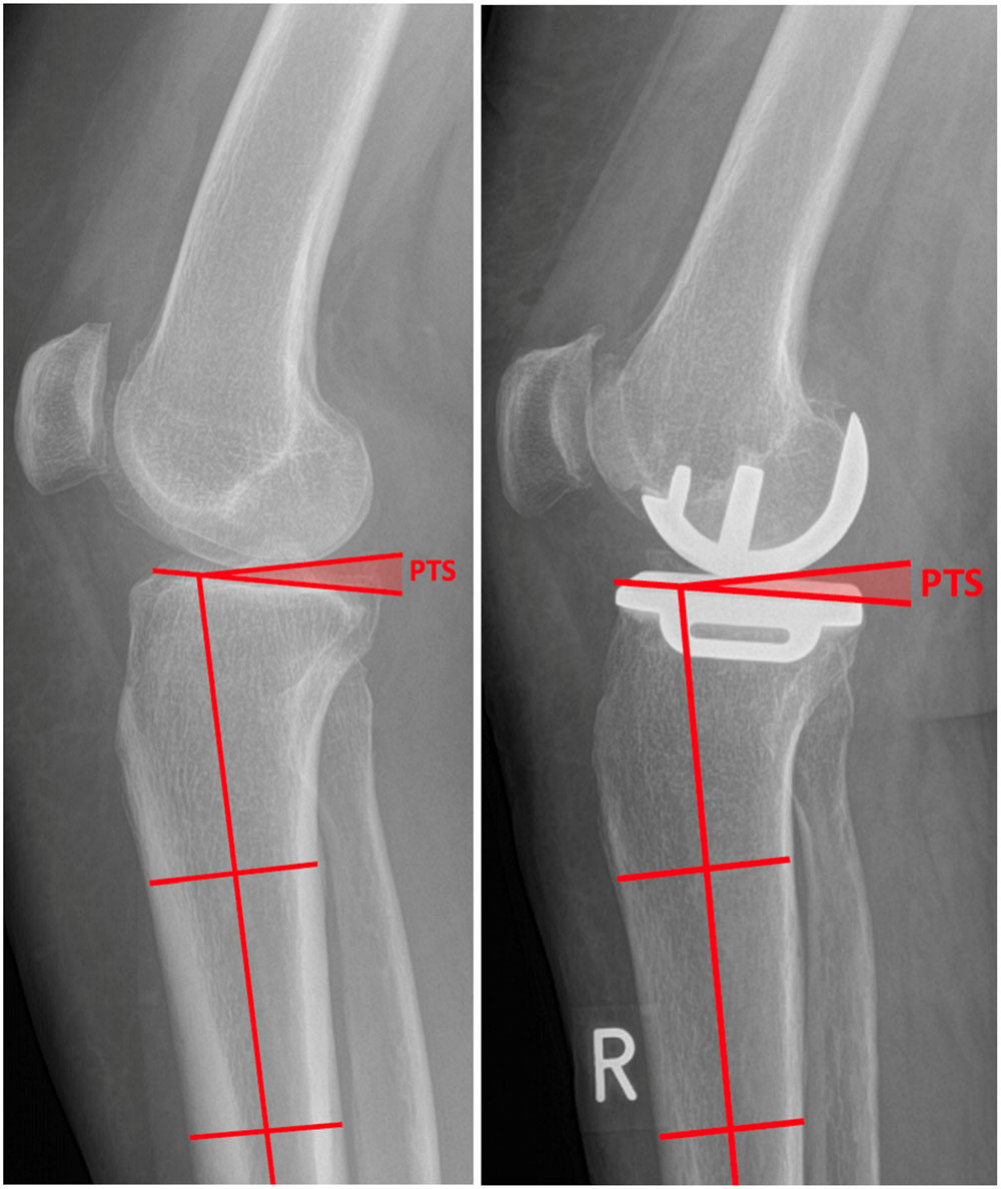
Measurements of mPTS. Left: mPTS lateral preoperative knee radiograph showcasing mPTS measurement. Right: mPTS lateral post‐operative knee radiograph showcasing mPTS measurement. mPTS, medial posterior tibial slope.
